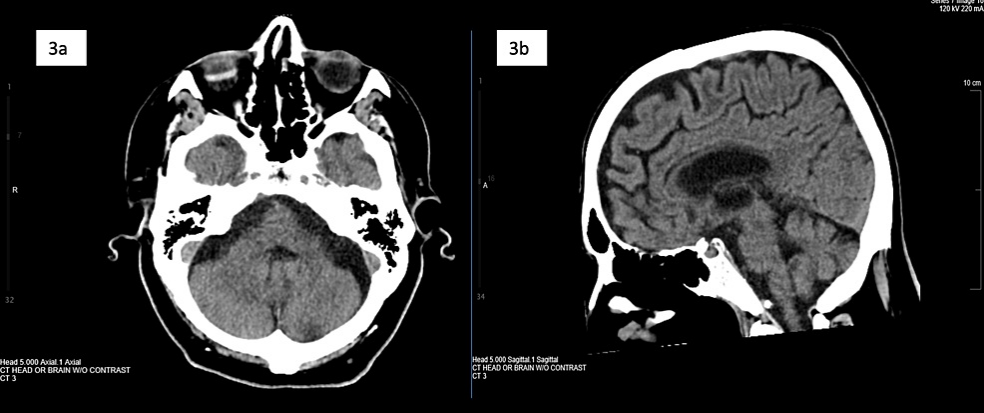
Repeat CT Scan Findings Showing Cerebellum and Brainstem, Axial and Sagittal Views
